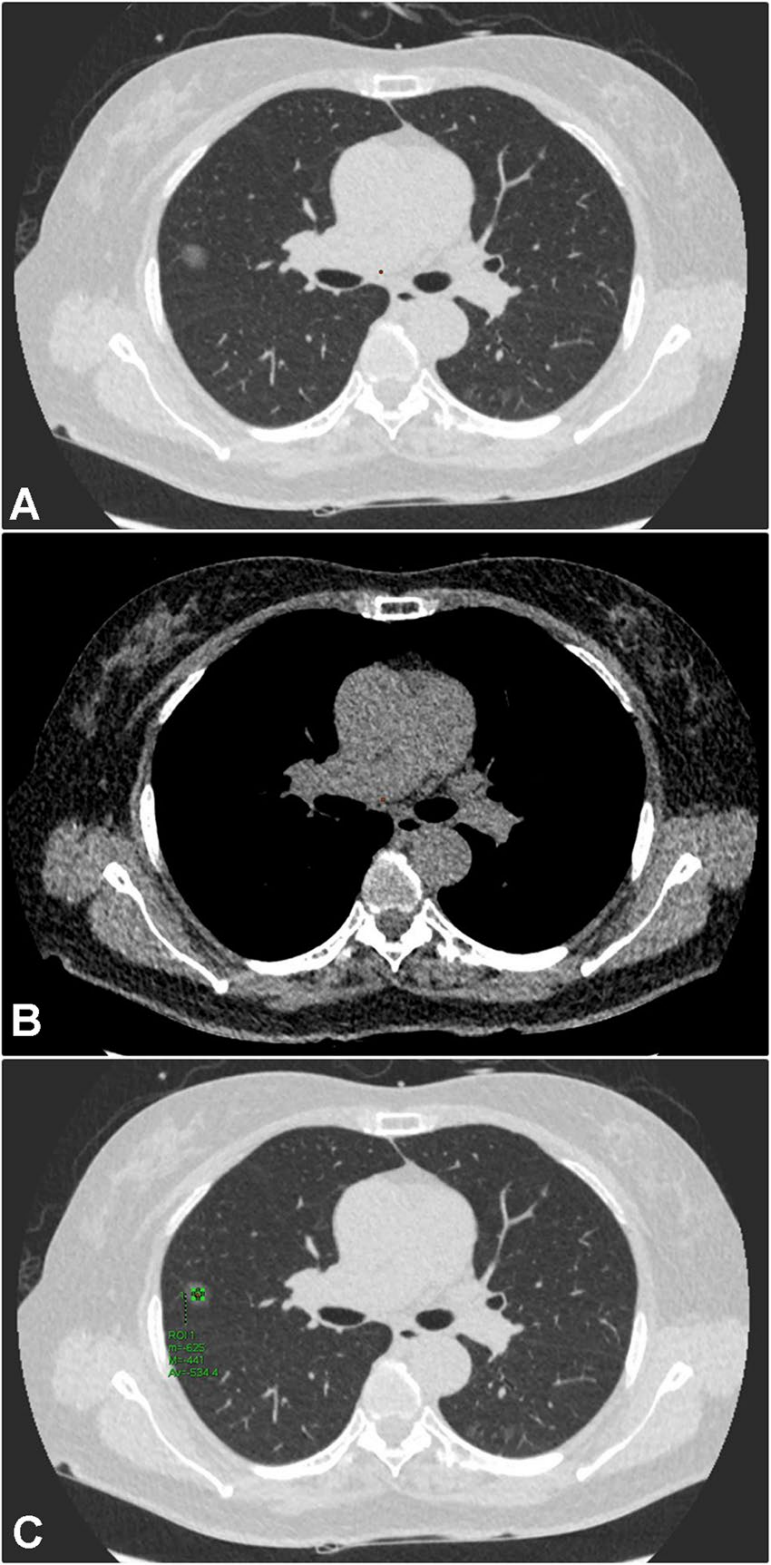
An example of pure GGN according to the SSN subclassification. A 60-year-old woman had a 1.3 cm pure GGN nodule in RML. The LDCT images showed homogenous groundglass opacities only when viewed on the lung window (Fig. 3A), but not seen on the mediastinal window (Fig. 3B). The average CT attenuation values (min, max and mean) expressed in HU were measured by placing a ROI of 15 mm^2^ on the lesion. In addition, to avoid placing the ROI box in or near the blood vessels could reduce the measurement errors (Fig. 3C). The patient underwent video-thoracoscopic wedge resection of RML. Further pathologic report demonstrated minimally invasive adenocarcinoma in RML. Abbreviations: GGN: groundglass nodule; SSN = subsolid nodule; RML = right middle lobe; ROI = region of interest; HU = Hounsfield unit.
